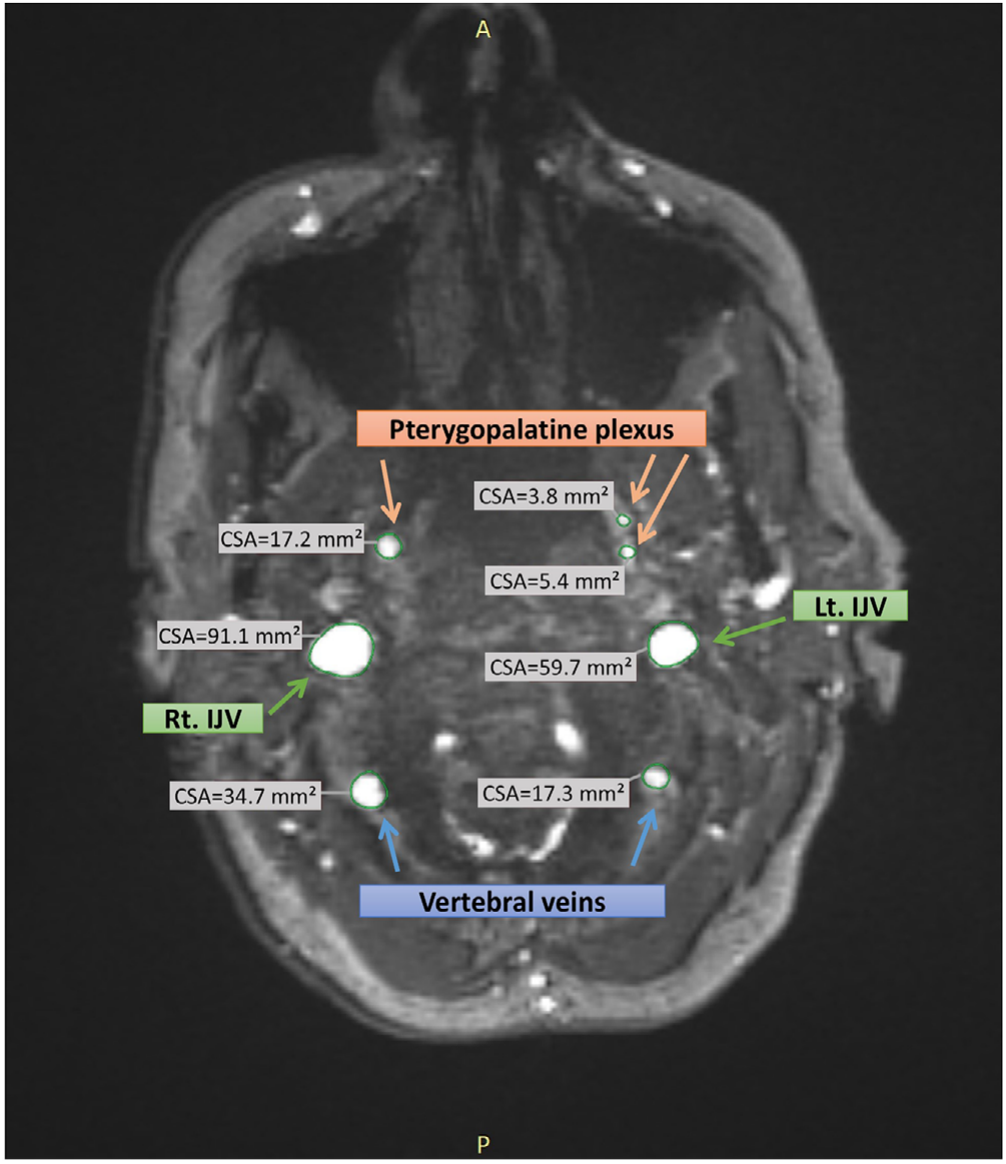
Measurement of cross‐sectional area using MRI time‐of‐flight sequence at level of jugular foramen. IJV, internal jugular vein; CSA, cross‐sectional area.
